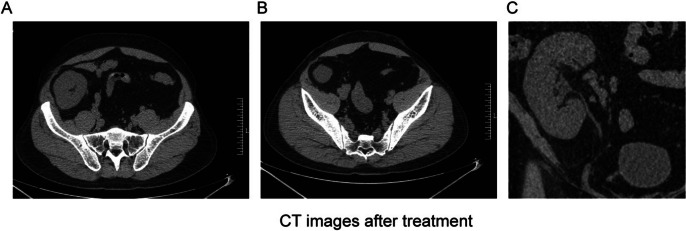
A. CT image showed that the transplanted kidney stone was completely cleared after 7 days treatment. B and C. CT image showed that ureteral calculus were completely cleared after 7 days treatment
